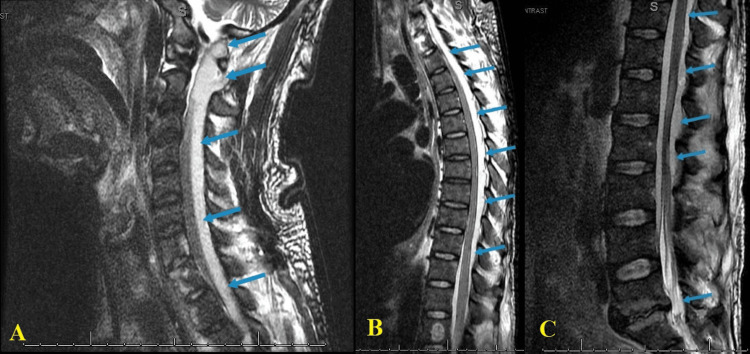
Holospinal epidural abscess T2-weighted sagittal MRI of the (a) cervical, (b) thoracic, and (c) lumbosacral spine demonstrating posterior, peripherally enhancing intraspinal epidural abscess (blue arrows) extending from the base of the skull to the sacrum (past the inferior margin of the scan: S1) MRI: magnetic resonance imaging
